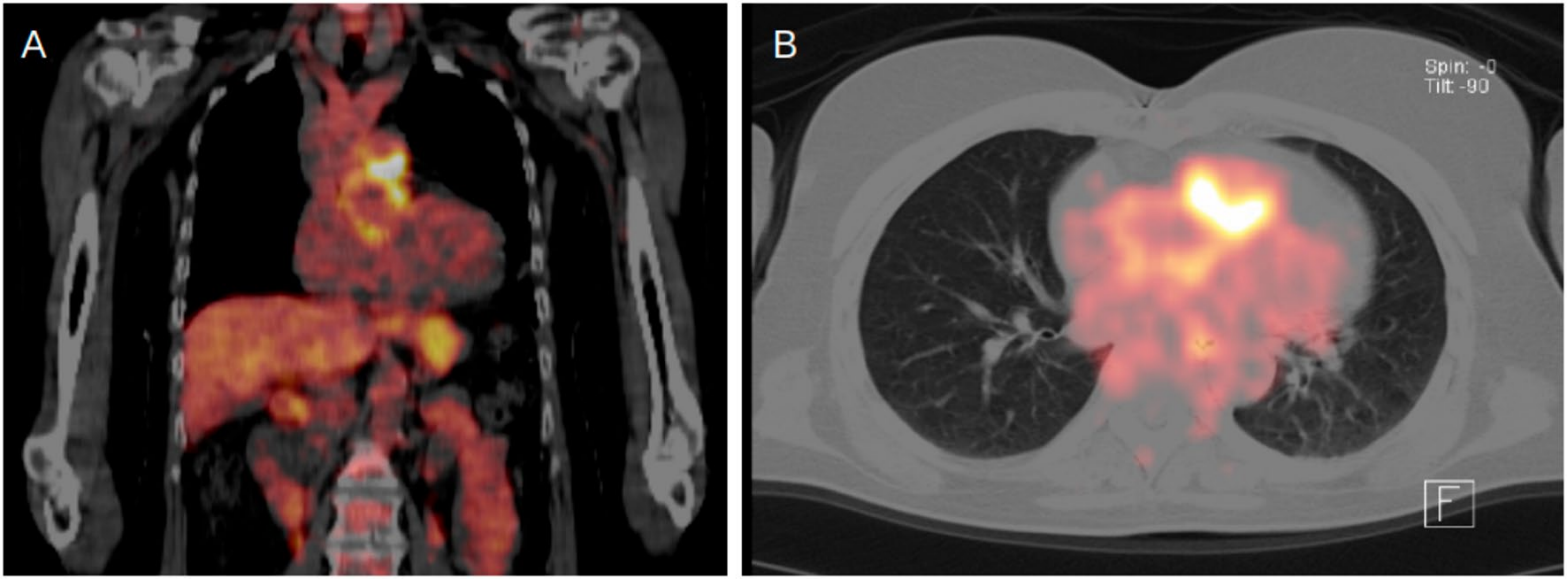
FDG-PET-CT scan indicating prosthetic graft infection in pulmonary position (frontal plane -A; horizontal plane -B), FDG-PET-CT - 18 F-fluorodeoxyglucose positron emission tomography-computed tomography
